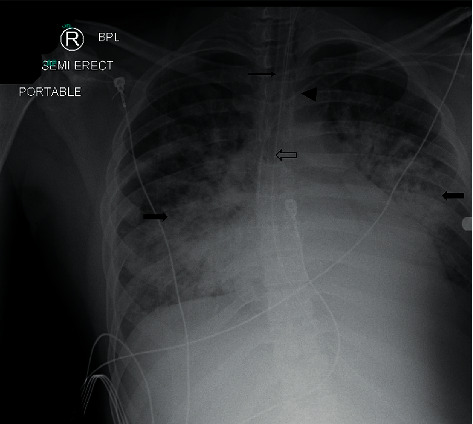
Chest X-ray obtained after endotracheal intubation showing extensive bilateral airspace consolidation (identified by block arrows with color fill) with some sparing of the apices. Tip of the endotracheal tube at the level of the clavicular heads (arrow). Nasogastric tube (block arrow without color fill) and right subclavian line (arrowhead) in place.
